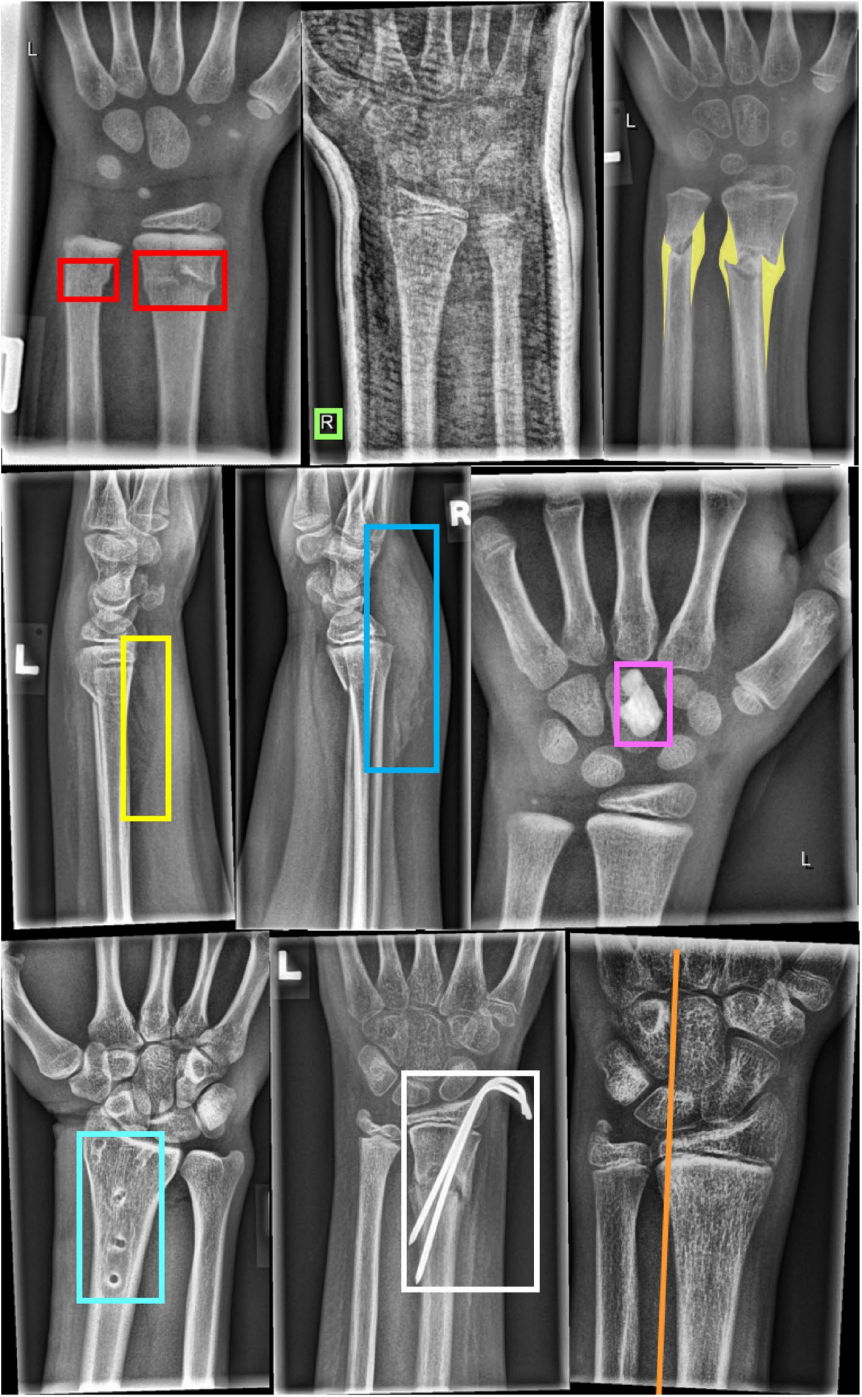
Examples of different objects labelled by the human experts. In the first row from left to right: Fracture (bounding box), text (bounding box), periosteal reaction (polygon). In the second row from left to right: Pronator quadratus sign (bounding box), soft tissue swelling (bounding box), foreign body (bounding box). In the last row from left to right: Bone anomaly (bounding box), metal (bounding box), and axis (line). The middle radiograph in the first row shows a cast. The right images in the first and third row were tagged with osteopenia.
